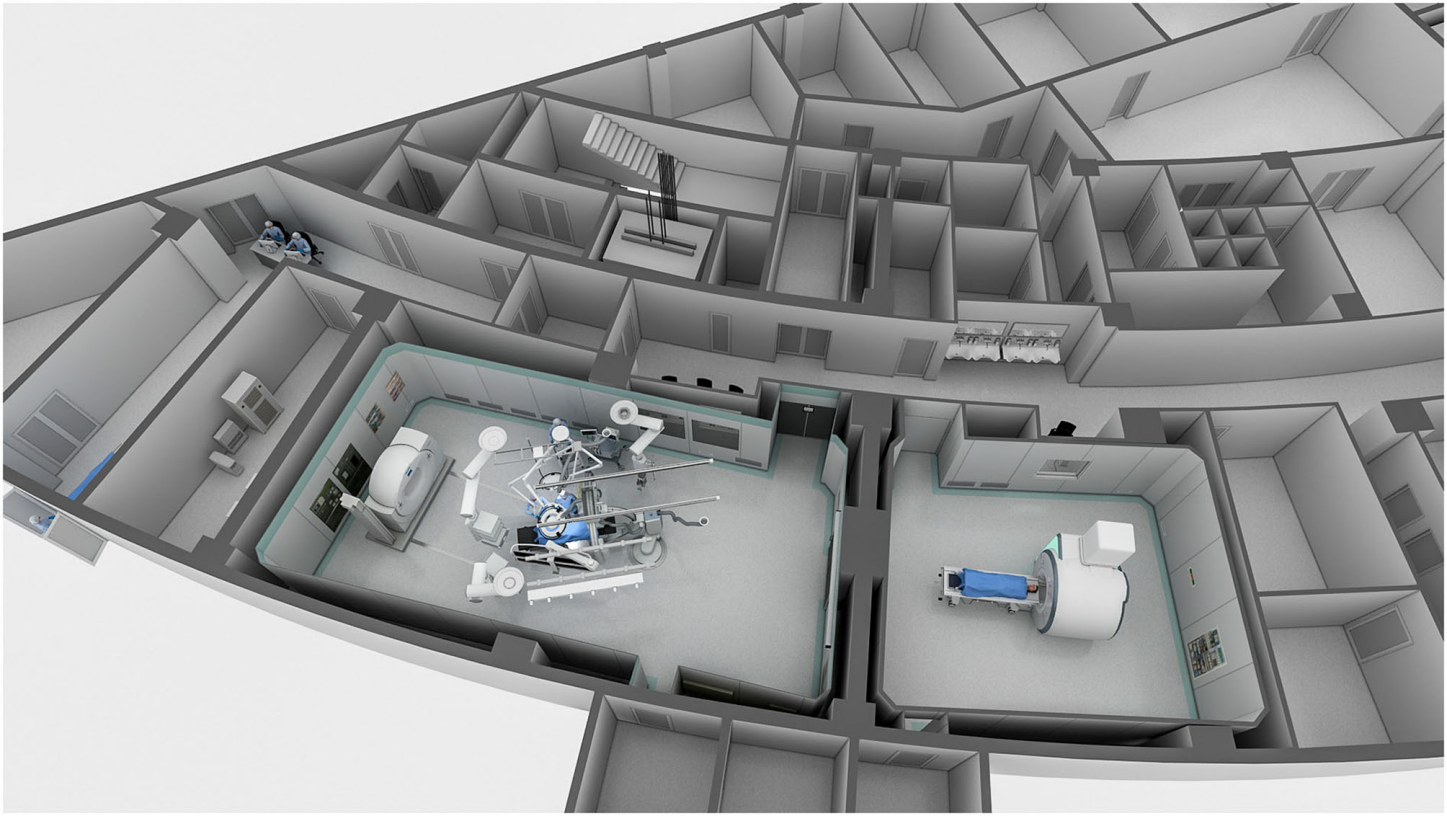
This overview of the one-stop stroke management (OSSM) platform shows the layout of the computed tomography (CT), magnetic resonance imaging (MRI), and digital subtraction angiography (DSA) equipment rooms.
